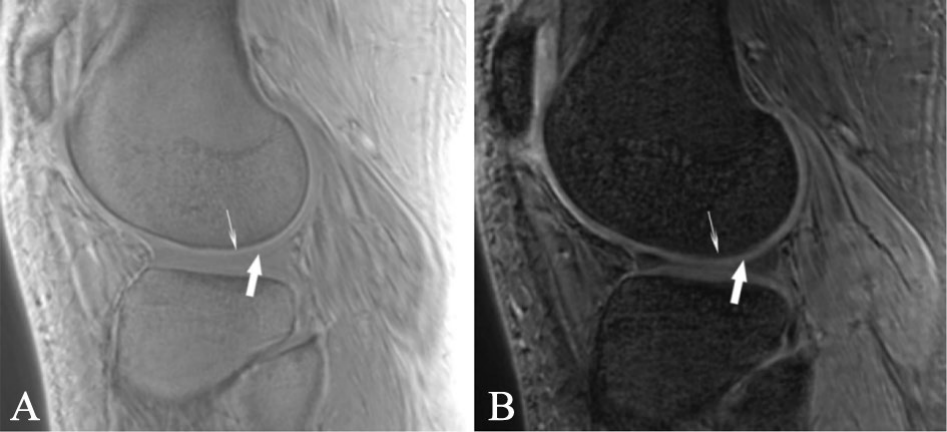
The appearance of cartilage on zero TE double-echo MR imaging. (A) On TE = 0 ms images, the calcified layer of the cartilage is a high-signal line (thin arrow) above the subchondral bone, while the other layers involved, including the superficial layer, the intermediate layer and the deep layer, are of intermediate signal intensity (thick arrow). (B) On TE = 4.6 ms images, the superficial layer, the intermediate layer and the deep layer of cartilage show high signal intensity (thick arrow), while the calcified layer of the cartilage and the subchondral bone are hypointense (thin arrow).
